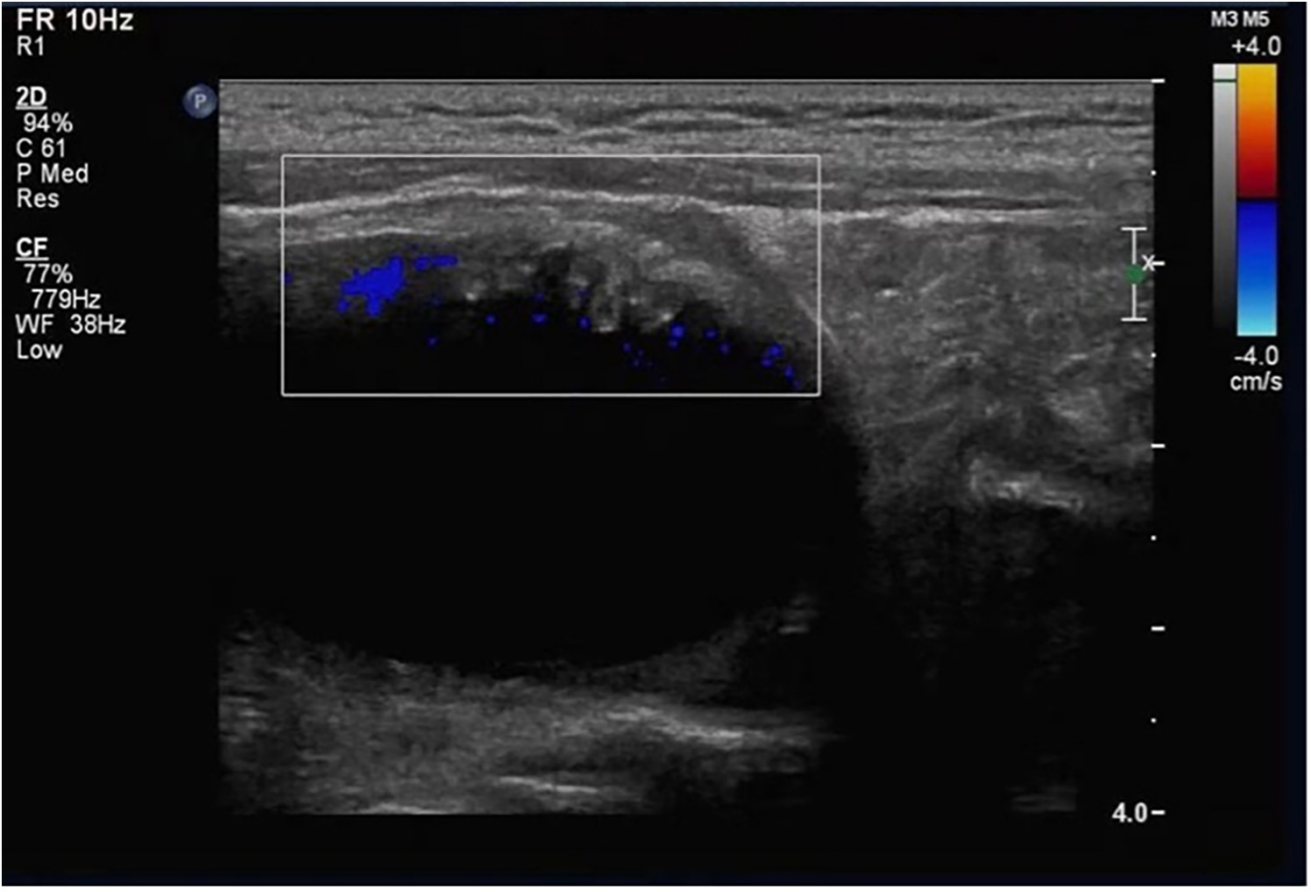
Ultrasound findings of the bladder wall. Localized papillomatous thickening of the bladder wall protruding into the bladder lumen, with visible vascular flow signal (color Doppler).
